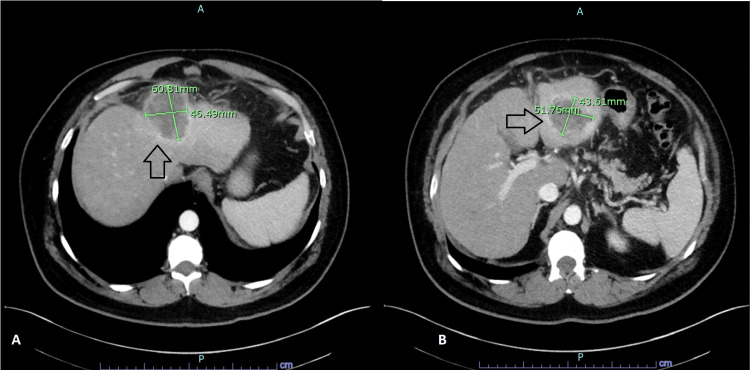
October 2019 CT scan of the abdomen There is evidence of two large left hepatic lobe masses (arrows in A and B)
